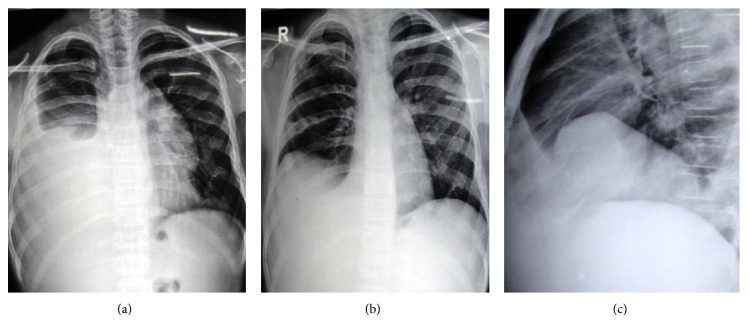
(a) Chest X-ray posteroanterior view showing right moderate pleural effusion with left mediastinal effusion (arrow). (b) Chest X-ray PA view postaspiration film showing residual right effusion and multiple osteolytic lesions in ribs bilaterally. (c) Chest X-ray right lateral view showing well defined opacity in lower part of chest.
